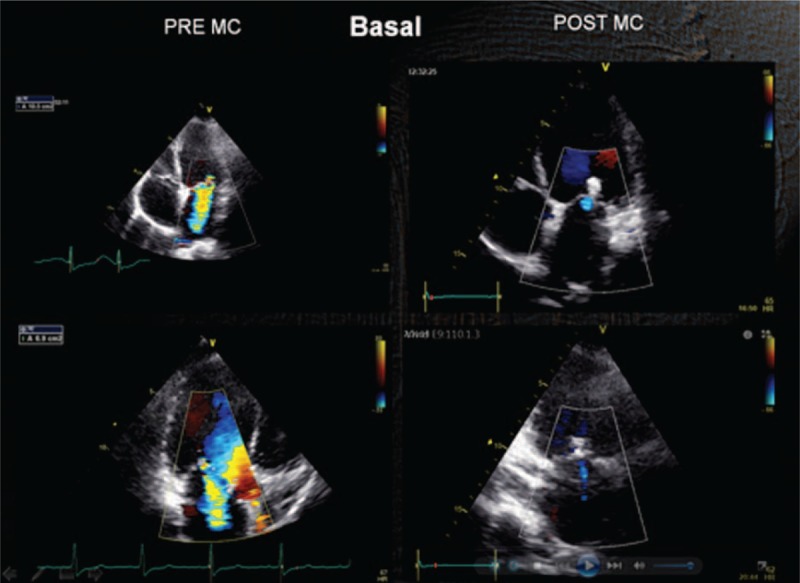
TTE at baseline preimplantation and postimplantation: after implantation regurgitation is almost absent. TTE = transthoracic echocardiogram.
